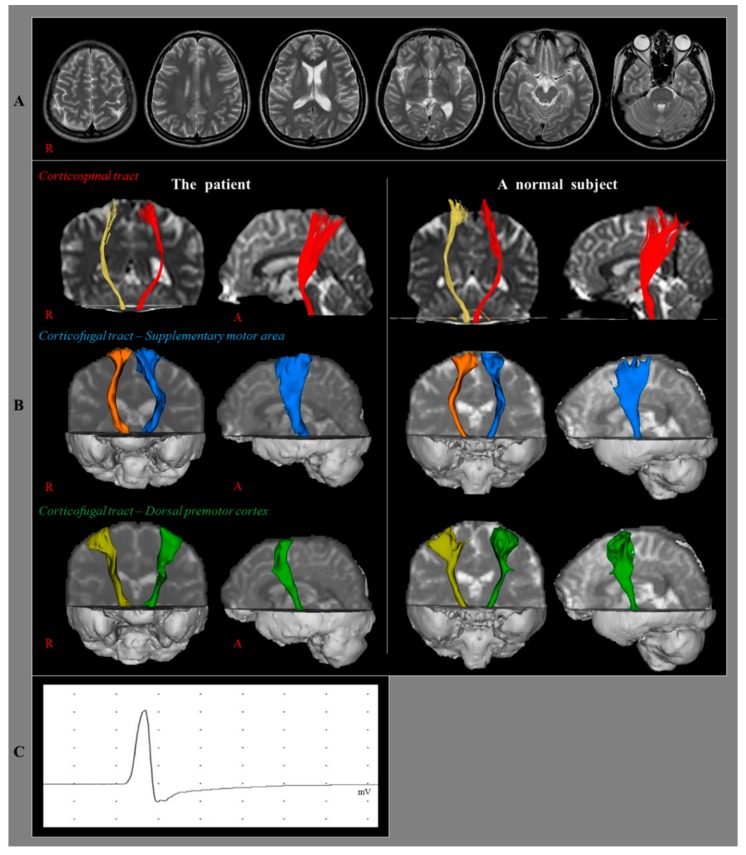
(A) Brain magnetic resonance imaging(MRI) images at 14 months after onset show no abnormal lesions. (B) Results of diffusion tensor tractography (DTT) for the corticospinal tract (CST) and corticofugal tract (CFT). On a 14-month DTT configuration, the left CST, CFT from supplementary motor area, and CFT from dorsal premotor cortex show similar configuration with a normal subject (24-year old female). (C) Results of transcranial magnetic stimulation (TMS). On a 14-month TMS study, motor evoked potential (MEP) is obtained at the right abductor pollicis brevis muscle during stimulation of the left hemisphere with 60% of maximal output (the MEP of shortest latency; 21.0 msec, amplitude: 5.1 mV).
